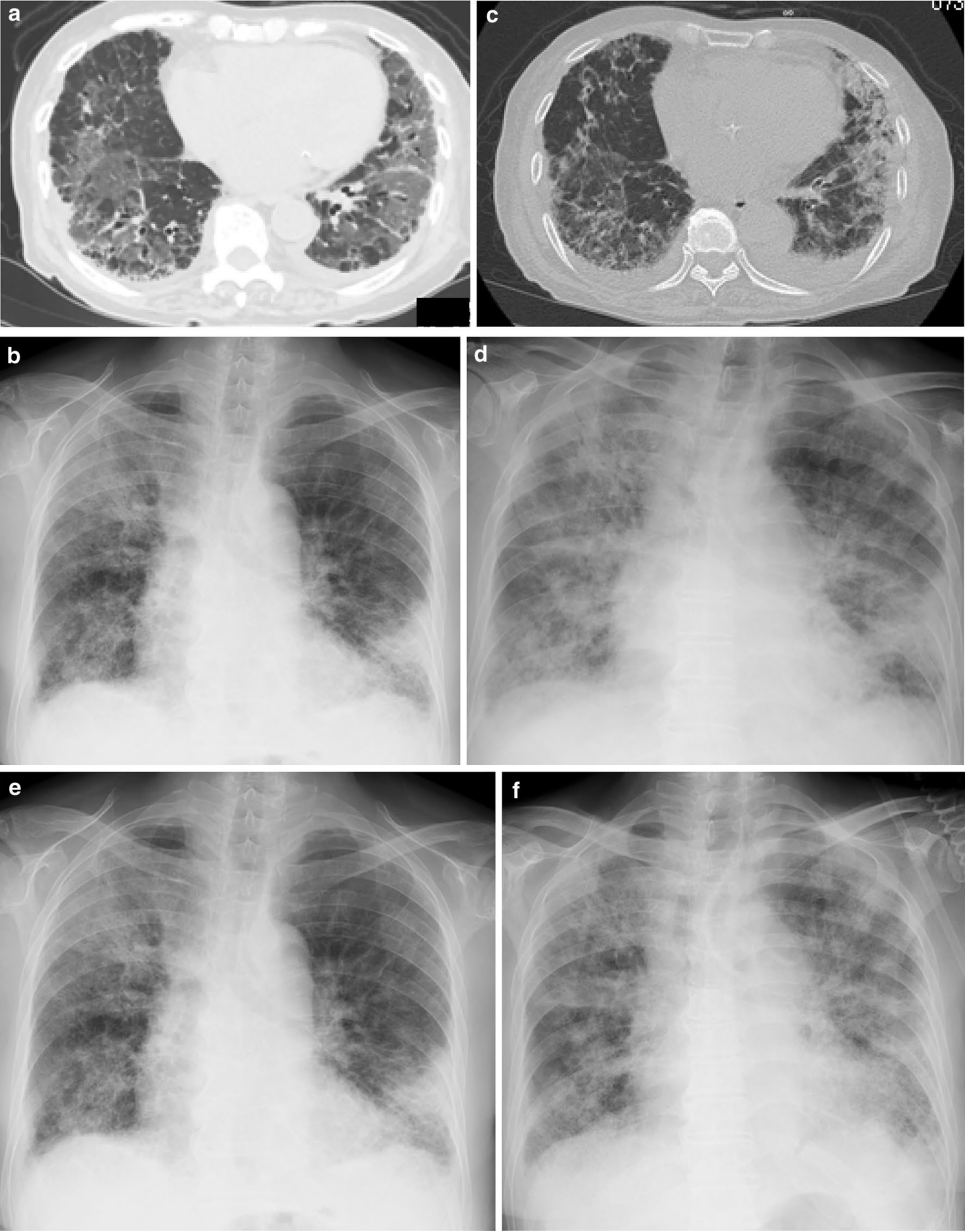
Chest CT and chest X-ray in the clinical course of the patient. a Chest CT showed that CT-attenuation of pulmonary infiltrates had increased and the beginning of architectural distortions was evident (second hospital day). b, c A chest X-ray and CT showed an increase in GGO and parenchymal consolidations with progression of the architectural distortions and pleural effusion (8th hospital day). d A chest X-ray showed an increase in GGO with pleural effusion (15th hospital day). e A chest X-ray showed no change in GGO with pleural effusion in spite of direct hemoperfusion using a polymyxin B-immobilized fiber column (18th hospital day). f A chest X-ray showed an increase in GGO with pleural effusion in spite of intravenous cyclophosphamide therapy (21st hospital day)
